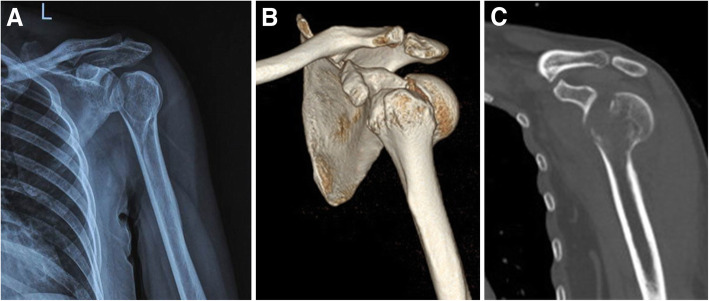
Case 2. A 45-year-old woman. AP X-ray (a) and 3-D CT (b, c) of the patient’s left shoulder immediately after injury demonstrating a fracture of the proximal humerus
